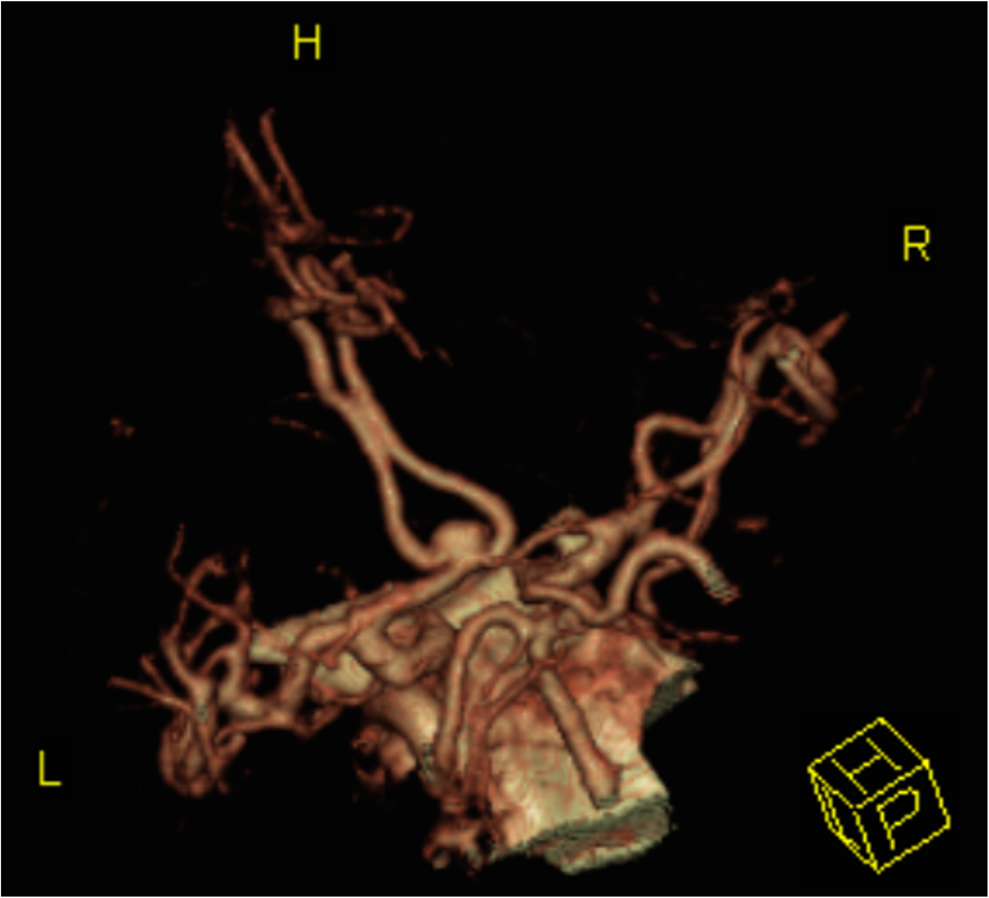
Computed tomography angiography image accompanying a case in the questionnaire, with the following text: “In your outpatient clinic, a 65-year old male presents with an anterior communicating artery (ACOM) aneurysm. The aneurysm has been incidentally discovered, and has a maximal diameter of 5 mm. The aneurysm wall is somewhat inhomogenous. The neuroradiologist informs you that endovascular treatment is possible. The patient is diagnosed with hypertension, but does not smoke and is otherwise healthy. He is willing to undergo prophylactic treatment, but is not particularly anxious about the risk of rupture in the case of abstaining from prophylactic procedures. Above is a CTA image of the aneurysm and surrounding vasculature.”
